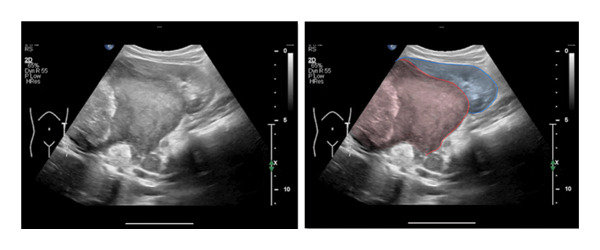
Renal Mass. Three‐years‐old girl with a palpable left flank mass. Ultrasound shows a mass (outlined in red) originating from the left kidney (blue). The mass is very inhomogeneous with calcifications and is compatible with a neuroblastoma.
